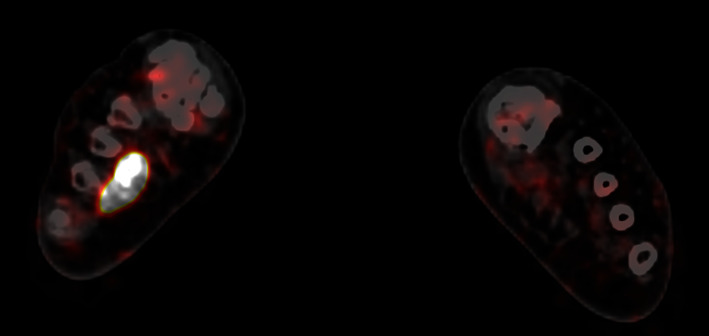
Bilateral feet ^68^Ga-DOTATATE-PET/CT images for Case 2. Axial ^68^Ga-DOTATATE-PET/CT slice through the bilateral feet demonstrating increased uptake in the right foot plantar soft tissue corresponding to the FGF-23 secreting PMT.
